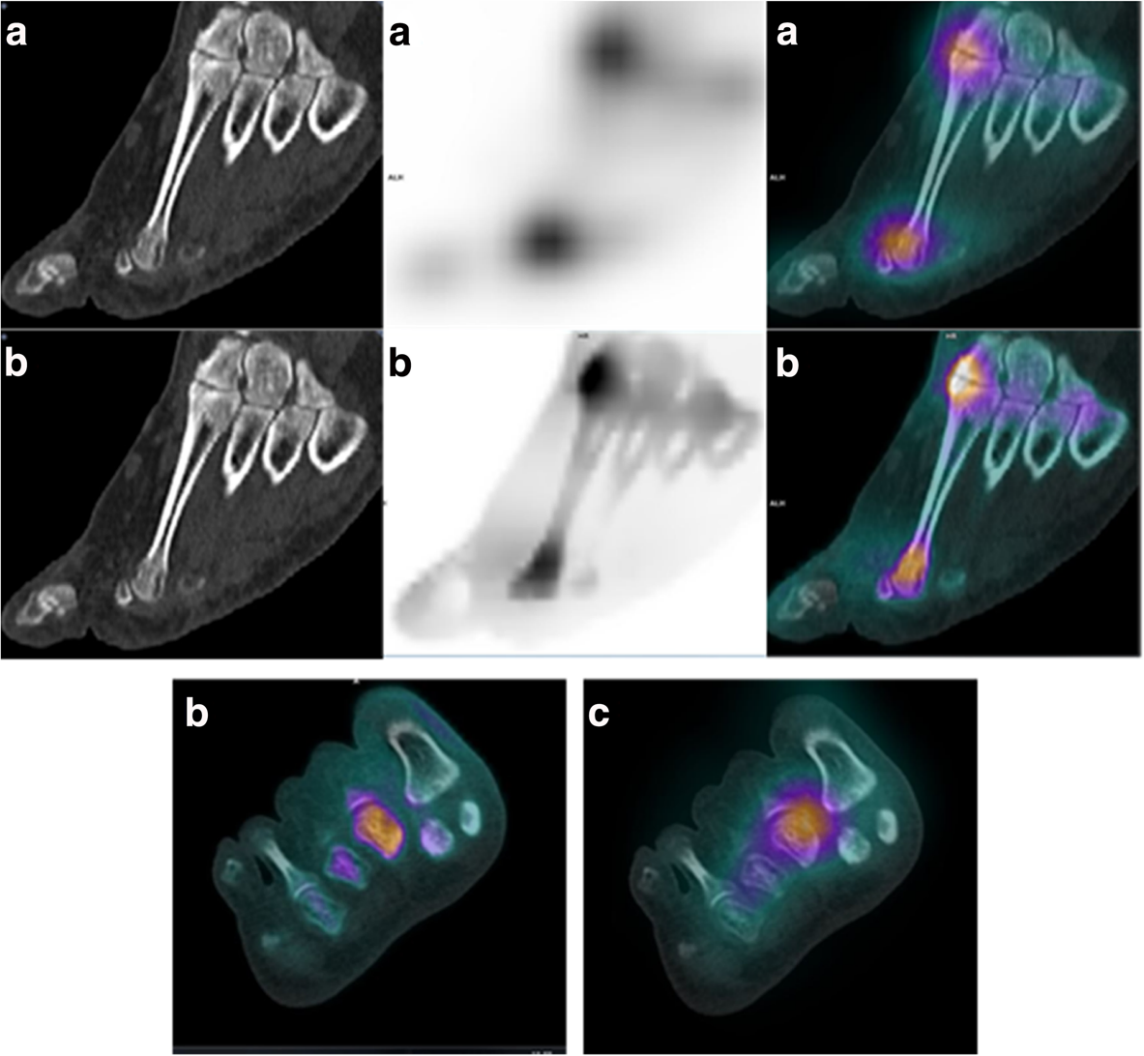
Location error of xSPECT Bone® related to the movements between SPECT and CT acquisition. This SPECT/CT was performed in order to explore a foot pain with suspicion of complex regional pain syndrome. An uptake of the second metatarso-phalangeal joint is observed on the F3D image (a) whereas an uptake of the head of the second metatarsal is observed on the xB image (b). The absence of traumatic context and the evolution with painful flares for 2 years suggests an osteoarthritic origin, confirming the hypothesis evoked by F3D/CT. Moreover, we can observe on the axial slice F3D/CT (c) a spatial shift between F3D acquisition and CT acquisition related to the movements of the patient, causing a bad reconstruction and a localization error of xB
